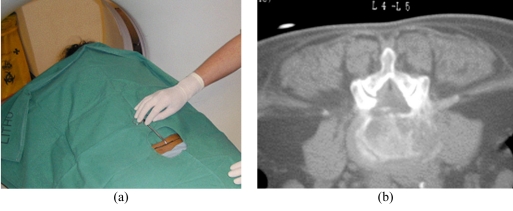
Free-hand method of surface marking for a lumbar biopsy. (a) Photograph shows the cleaned and draped patient lying in a prone position. A sponge forceps is used to indicate the estimated skin puncture point. (b) CT fluoroscopic image taken with the patient lying prone shows the tip of the sponge forceps at the proposed needle entry point. A right posterolateral paravertebral approach was planned for biopsy of the L4/5 disc.
